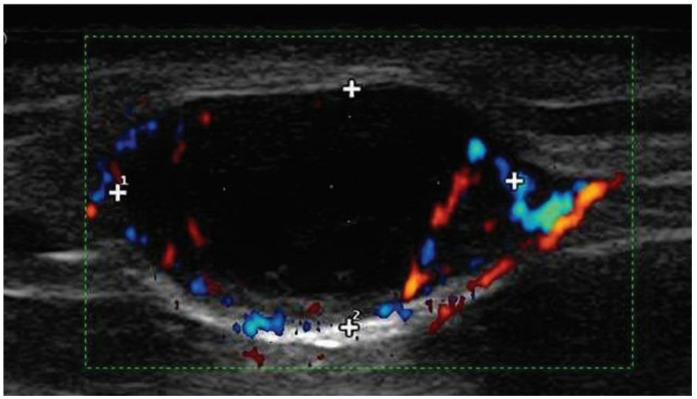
Doppler ultrasound image of a lymph node demonstrating the three key features identified by Dr. Voit in determining survival predictability: (1) loss of central echos, (2) peripheral perfusion and (3) balloon shaping. (Image courtesy of the late Prof. Dr. Christiane Voit).
